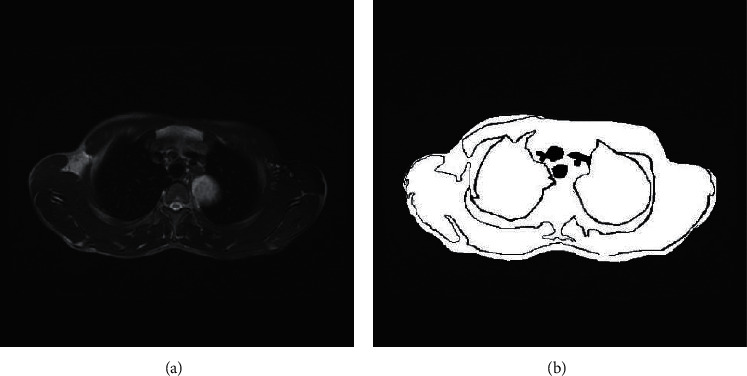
Comparison of MRI images of lung tumors before and after binarization. (a) Original MRI of pulmonary tumors. (b) MRI after lung tumor binarization.
